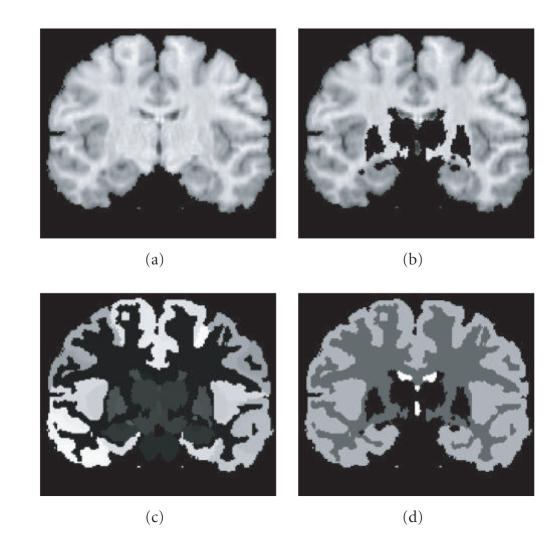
MRI brain data. (a) Original slice with cortical
